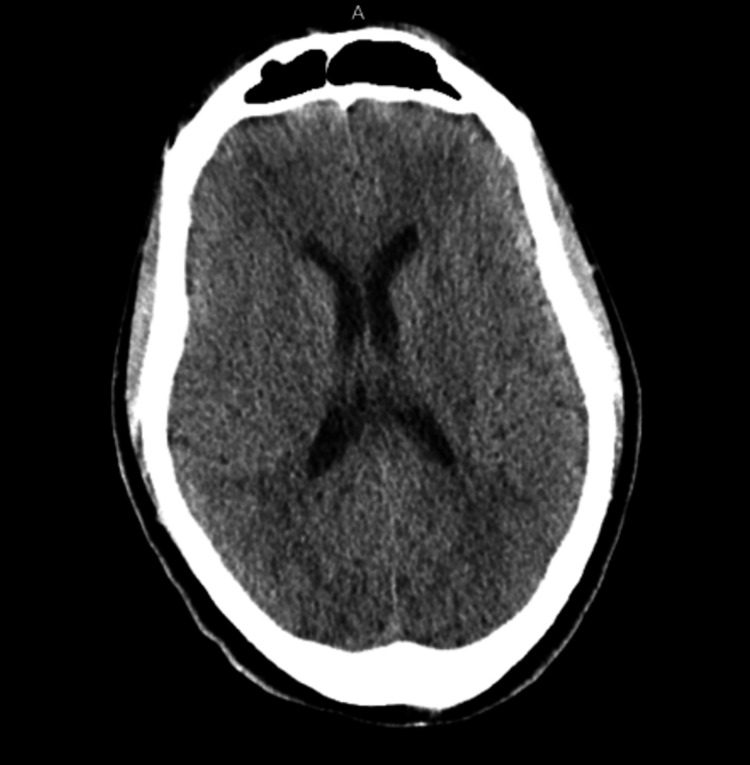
CT of brain without contrast showing no acute intracranial pathology. Ventricular and sulcal size and configuration were within normal limits. No acute loss of gray-white differentiation was noted, nor was there any acute intracranial hemorrhage or extra-axial collection. There was no evidence of mass effect or midline shift. The basilar cisterns were patent. There was no acute displaced calvarial fracture. The tympanomastoid air cells, along with the visualized portions of the orbits and paranasal sinuses, were within normal limits. CT: Computed tomography
